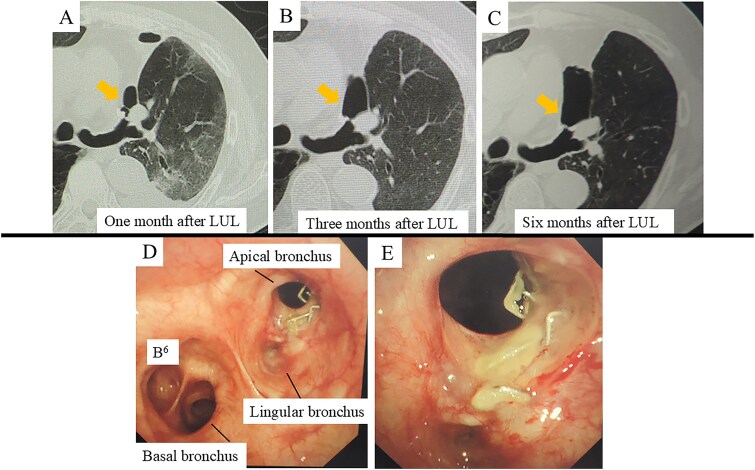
CT and bronchoscopic findings post-left upper lobectomy (LUL). (A–C) CT scans obtained at 1, 3, and 6 months postoperatively. Panel A shows a small airspace (gold arrow) near the upper segment of the bronchial stump that progressively enlarges over time. (D–E) Bronchoscopic images at 6 months demonstrate failure of the staple line for the upper division bronchus (B1 + 2, B3) at the upper lobe bronchial stump, resulting in an ~10 mm complete separation with surrounding whitish changes.
